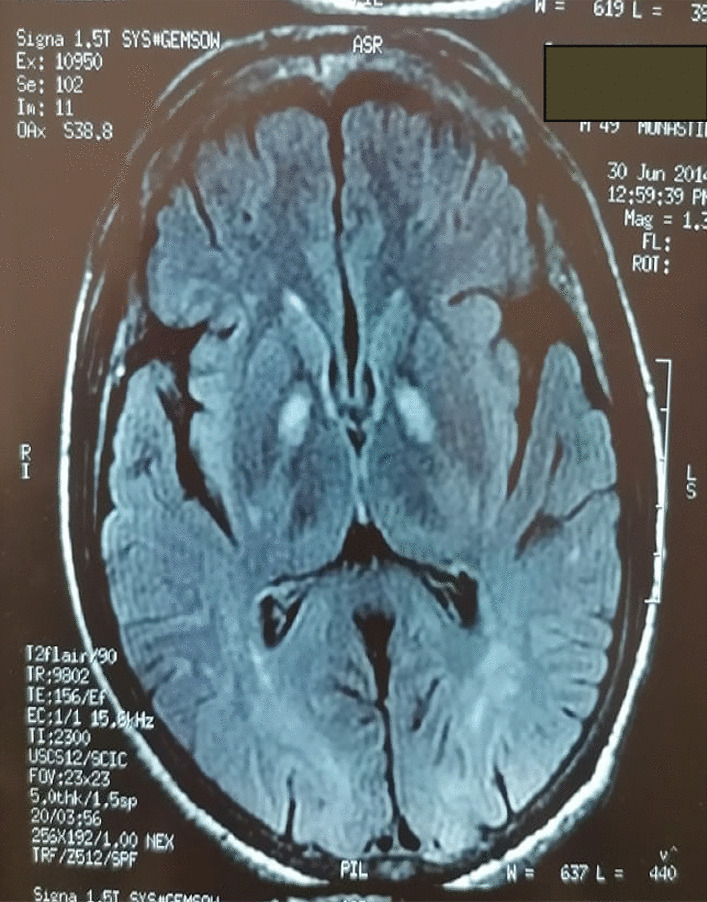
Cerebral magnetic resonance imaging: axial slice, seq T2 Flair: bi-pallidal hypersignal
